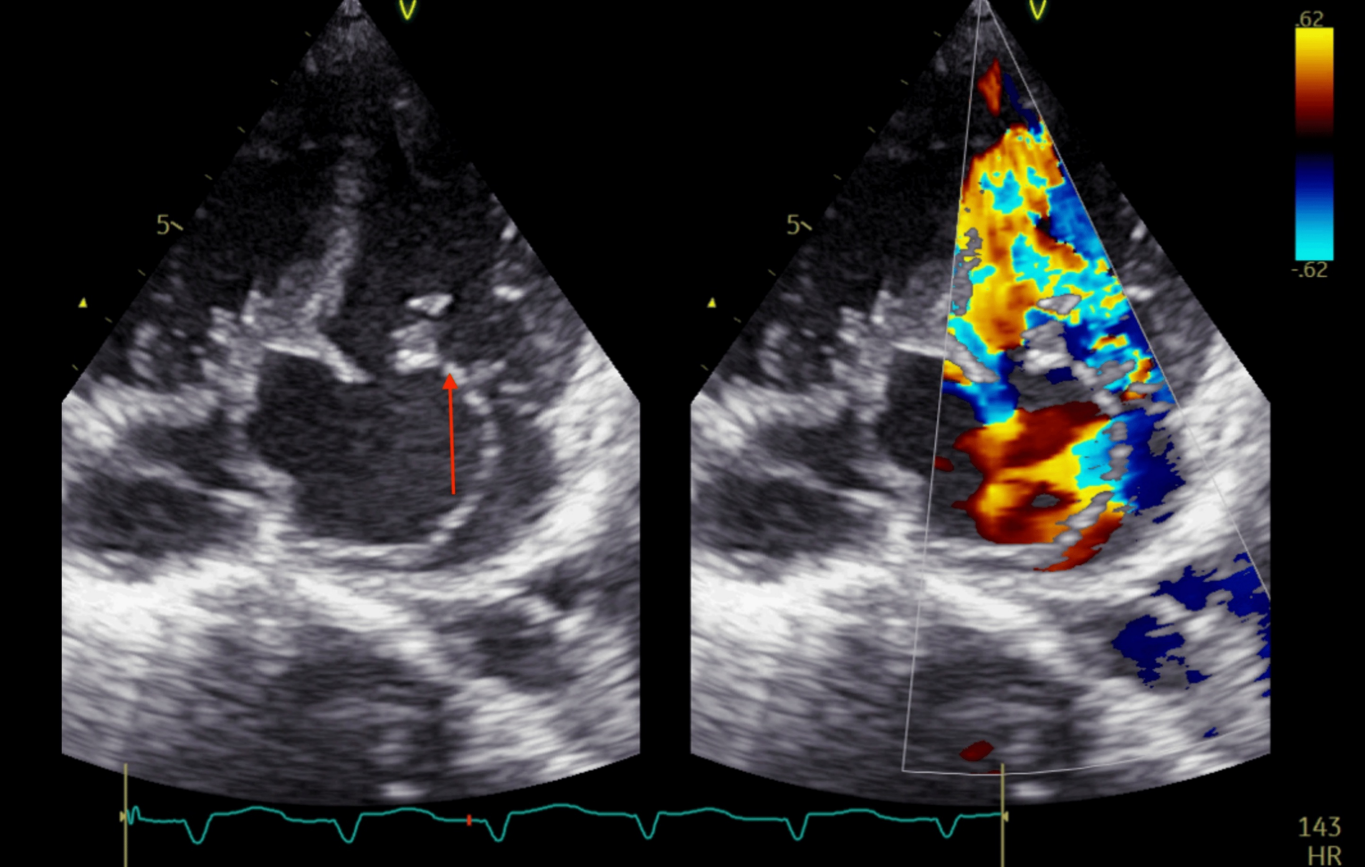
Transthoracic echocardiography showing anterior mitral leaflet perforation (red arrow) with severe mitral regurgitation demonstrated on color Doppler
